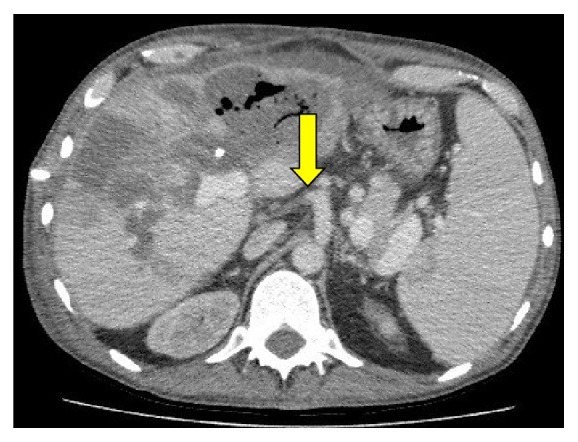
CT scan in a patient following liver transplantation who developed liver abscess. The CT scan demonstrated an occluded transplanted hepatic artery (HAT) arising from the celiac artery (yellow arrow).
